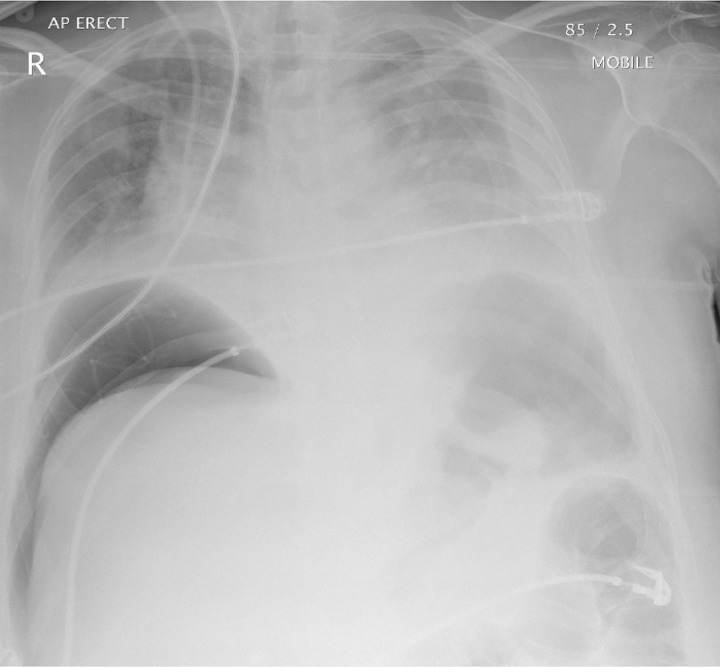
Erect chest x-ray confirming perforation following withdrawal of the nasogastric tube
